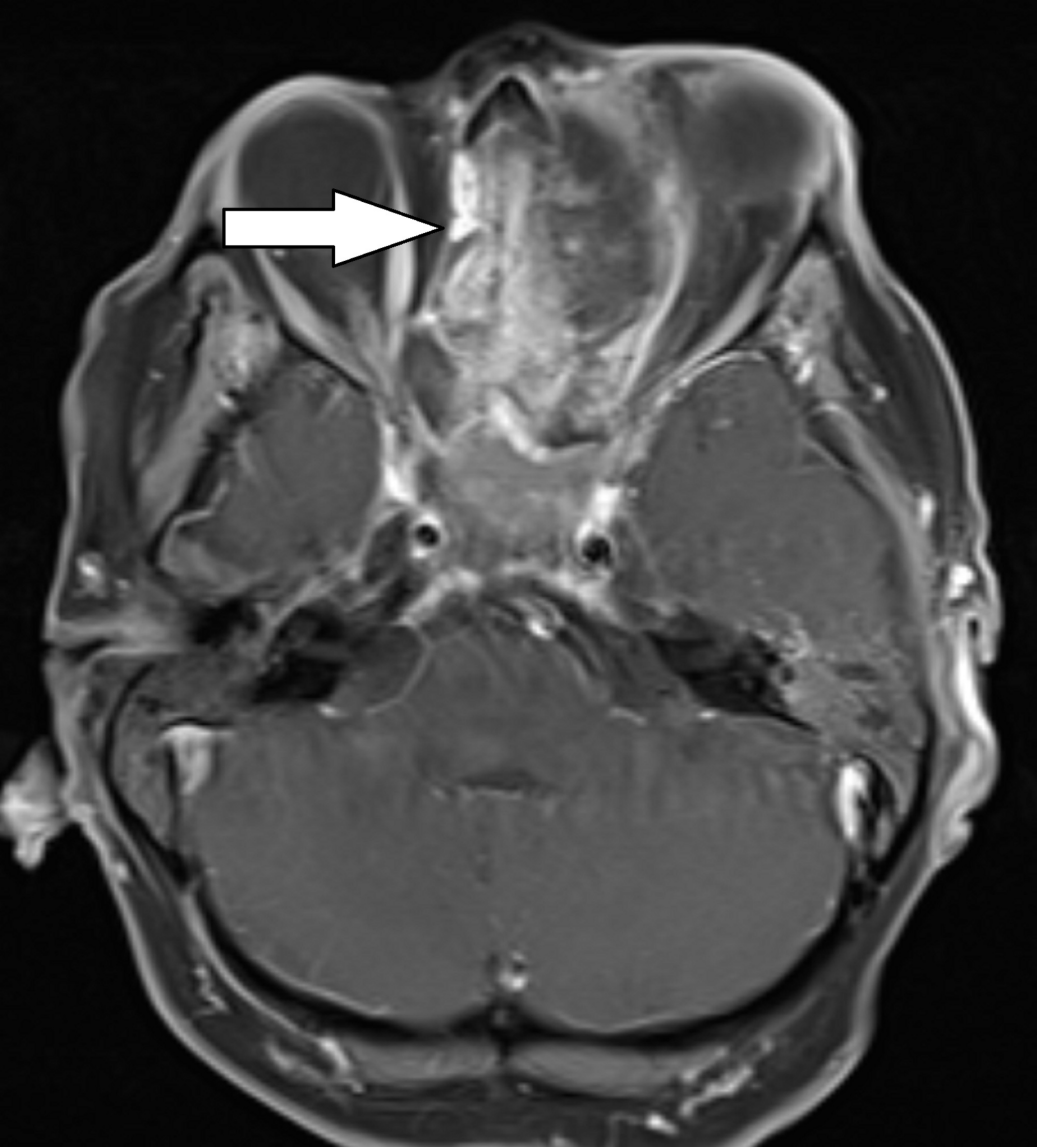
T1 weighted post-contrast axial MRI
